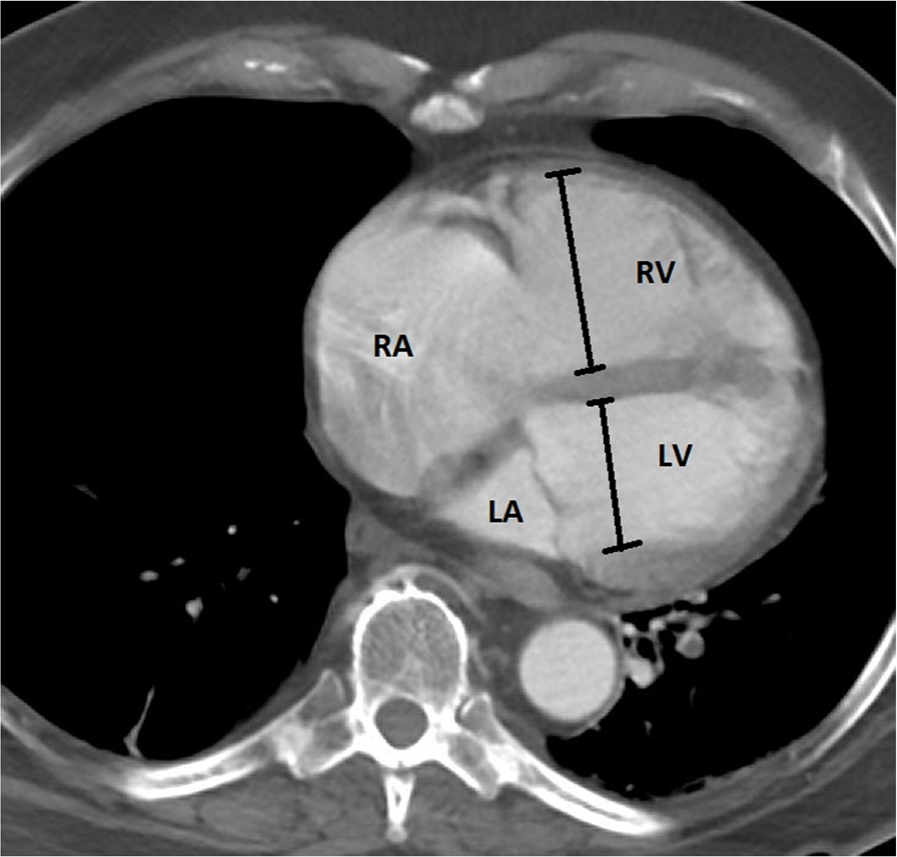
Measurement of RV/LV ratio. Axial CT images demonstrate the best approximated four-chamber view. Calipers are placed from inner wall to inner wall at the maximal diameter on a view that best approximates the four-chamber view
